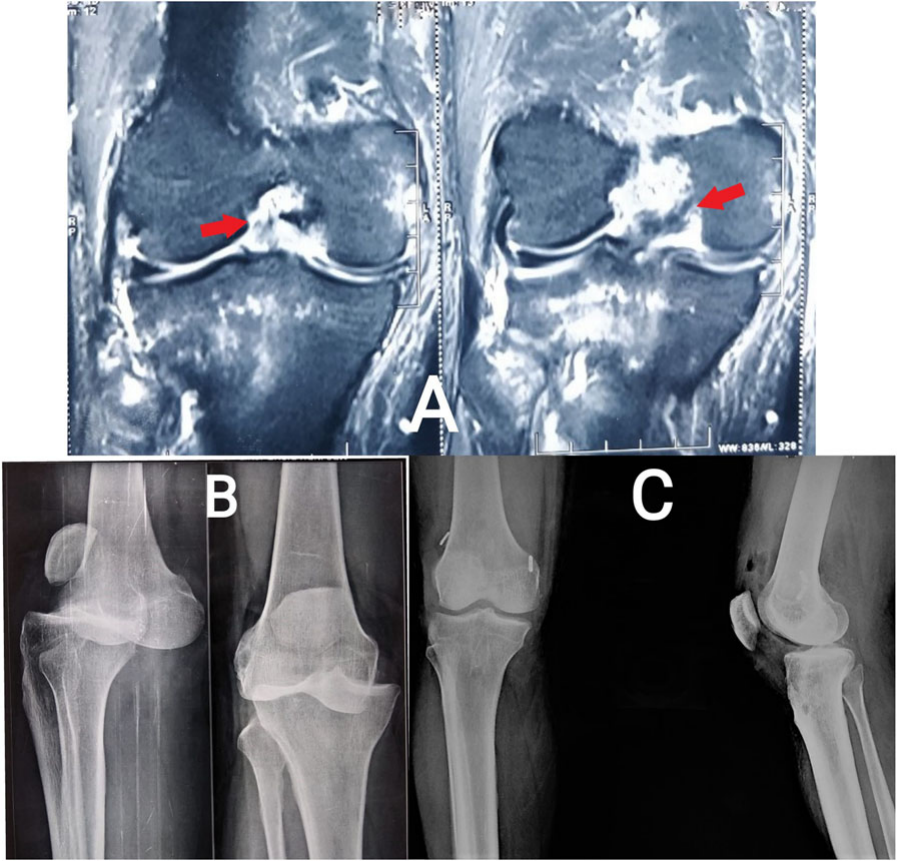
a Fat-suppressed coronal MRI images of a patient with ACL and PCL tears (*red arrows* show discontinuity of fibres from the femoral attachments). b For patient with a knee dislocation, radiographs before reduction of the knee joint. c For patient with a KD type II injury, postoperative radiograph after ACL and PCL reconstruction
